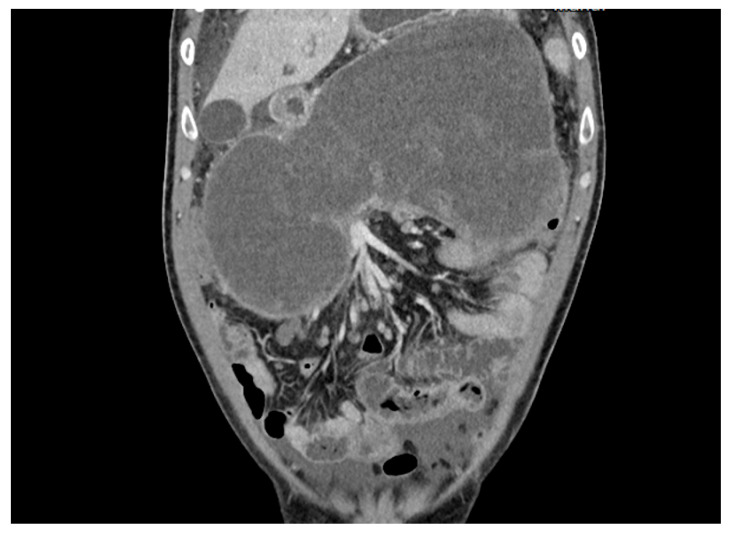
CT scan of a 35-year-old patient with a pancreatic pseudocyst.
